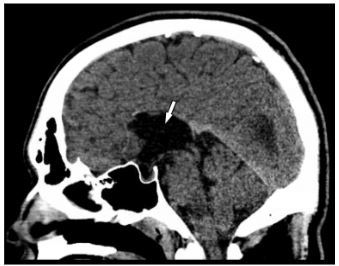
Sagittal view of computed tomography of the head demonstrating complete agenesis of the corpus callosum (arrow).
